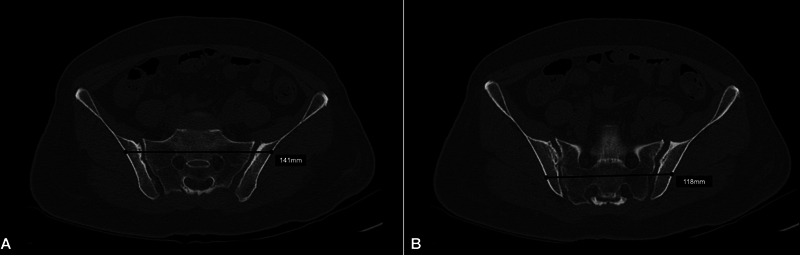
Case example (preoperative planning). Axial computed tomography scans demonstrating the measurement of the length of the bone corridors for fixation in the first (A) and second (B) sacral segments during preoperative planning.
